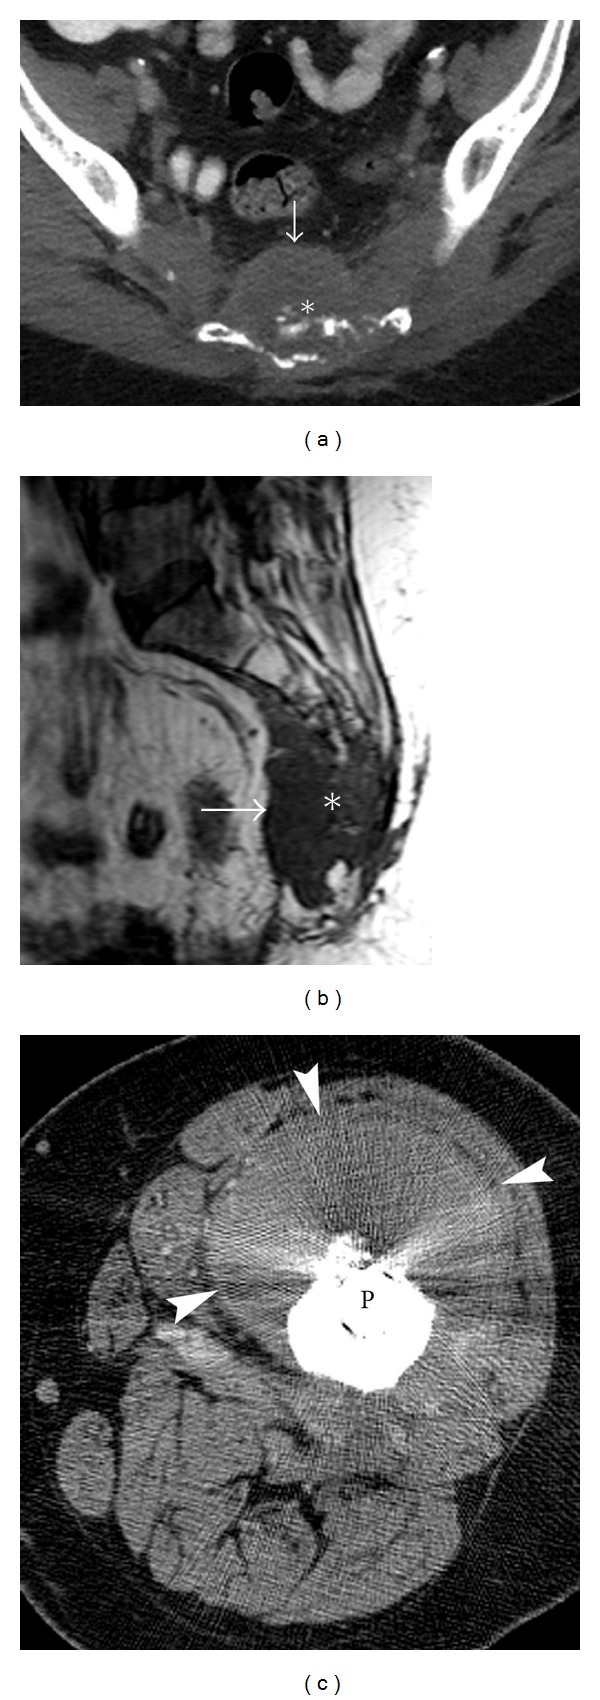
Chordoma. A 64-year-old female presenting with low back pain, diagnosed with an advanced sacral chordoma. Axial CT (a) and sagittal T1 midline image (b) demonstrate a large midline mass with internal calcification, destroying the sacrum (asterisk) and infiltrating the presacral space anteriorly and epidural space posteriorly. Axial CT (c) showing a large soft tissue metastasis (arrowheads) in the thigh around the femur. Hardware artifact is from an intramedullary rod (P) internally fixing the pathological fracture of the femur.
